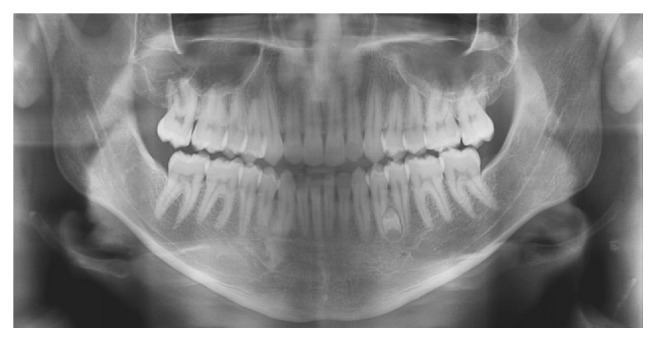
Panoramic radiograph of the patient aged 20 years and 5 months showing a supplemental supernumerary tooth in the left mandibular premolar region.
